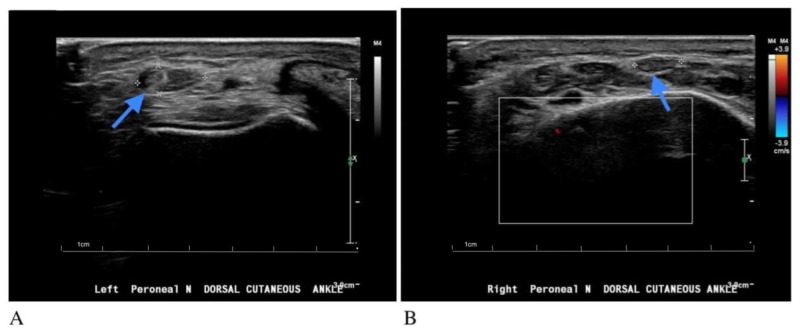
Images of neuromuscular limited ultrasound (Phillips, Affiniti 50G) using high frequency transducer (7-14 MHz). Image A shows enlarged left DCPN with cross-sectional area of 27.88 mm^2^ as compared to Image B which shows right DCPN with cross-sectional area of 10.8 mm^2^. DCPN: Dorsal cutaneous peroneal nerve.
